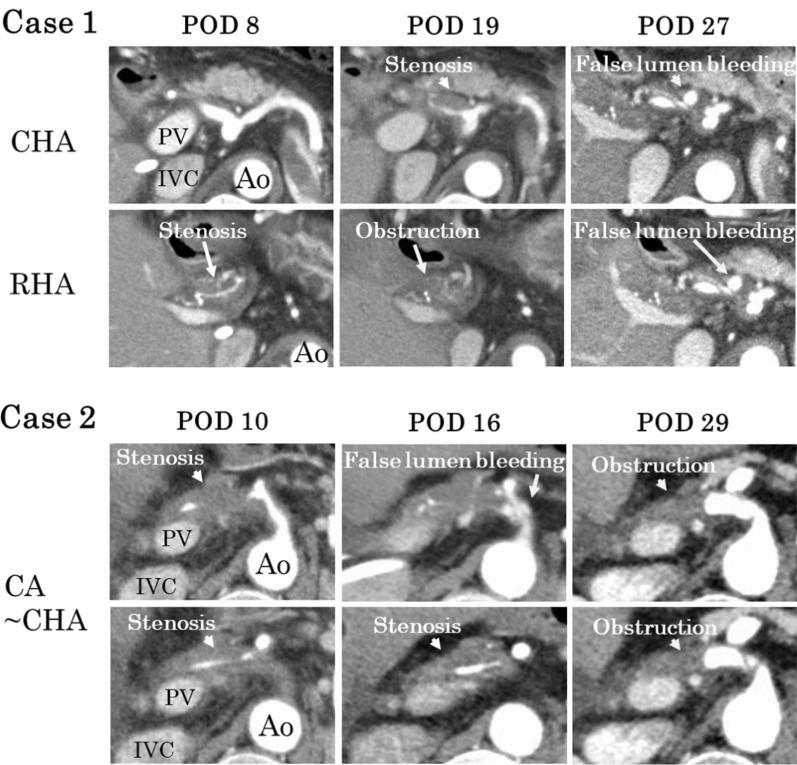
Serial contrast-enhanced CT for Patient 1 and Patient 2. Case 1: Contrast-enhanced CT on postoperative day (POD) 8, 19, and 27 for Patient 1. Contrast-enhanced CT on POD 8 reveals stenosis of the right hepatic artery (RHA) and a low-density area around the common hepatic artery (CHA), suggesting dissection with the formation of a false lumen. There was no bleeding in the false lumen. On POD 19, an obstruction of the RHA and stenosis of the CHA was observed. On POD 27, bleeding in the false lumen was observed around the CHA and the celiac artery (CA). Case 2: Contrast-enhanced CT on POD 10, 16, and 29 for Patient 2. Contrast-enhanced CT on POD 10 reveals a stenosis of the CHA and the CA as well as a low-density area around them. On POD 16, bleeding in the false lumen was observed around the CA. On POD 29, an obstruction of the CHA was observed, and the bleeding in the false lumen around the CHA and the CA increased chronologically. Abbreviations: PV, portal vein; IVC, inferior vena cava; Ao, aorta
